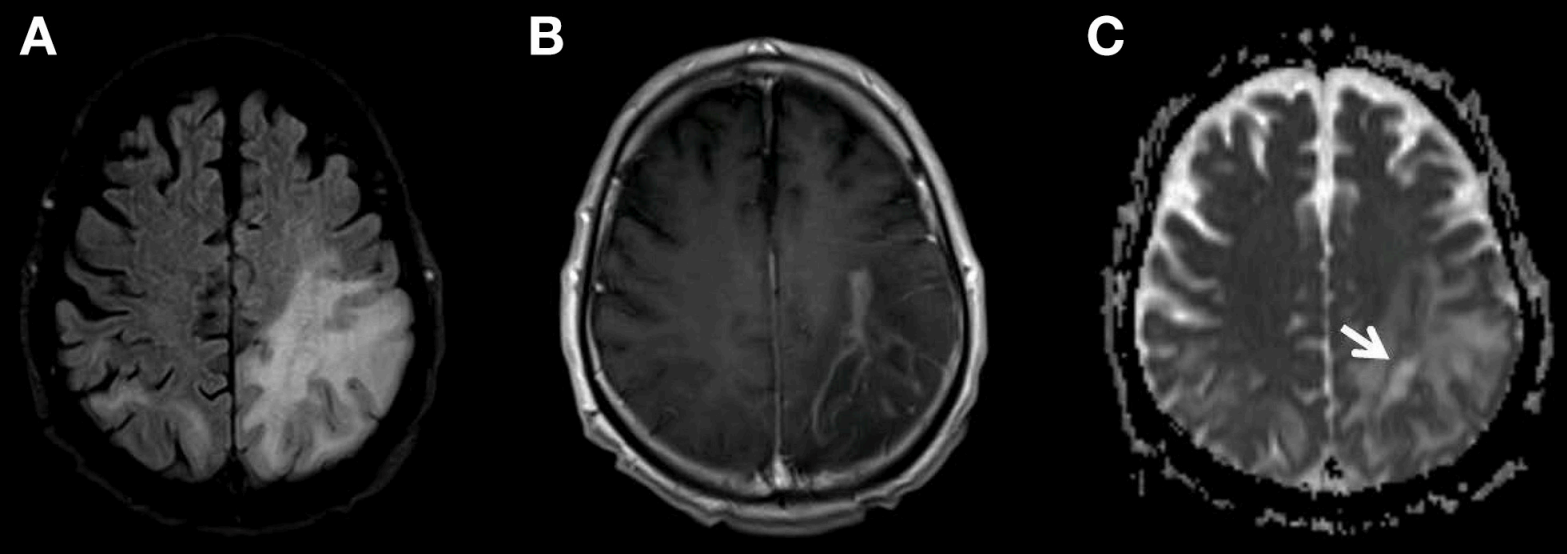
MR examination showing the progress of the PML-related FLAIR-hyperintensities (A). The new multifocal and strong contrast enhancement (B) indicates the PML-IRIS. The zone of active inflammation and demyelination is again indicated also by the low ADC-rim surrounding the area affected by the JC-virus (C, white arrow).
